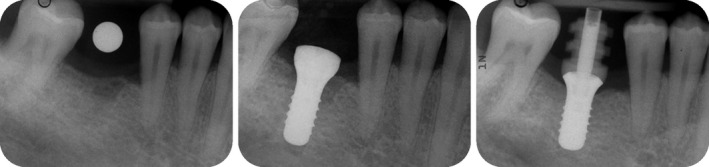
Radiographic planning for implant placement in the area of 46
